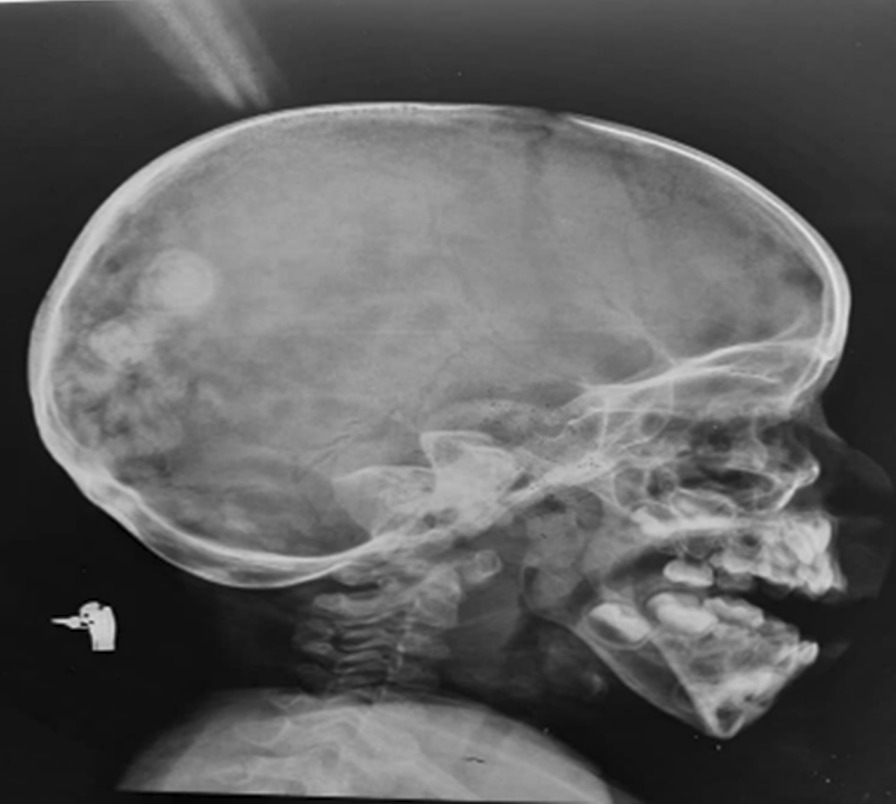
X-ray shows subcutaneous calcifications over the skull
